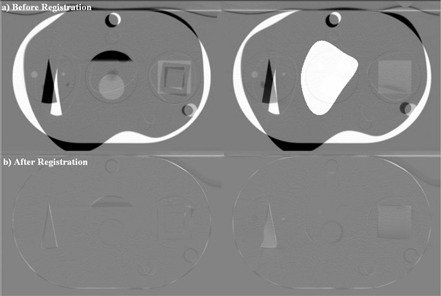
Subtraction images of a computed tomography slice (a) before and (b) after registration to the artificially deformed computed tomography slice with deformations ±50 mm.
